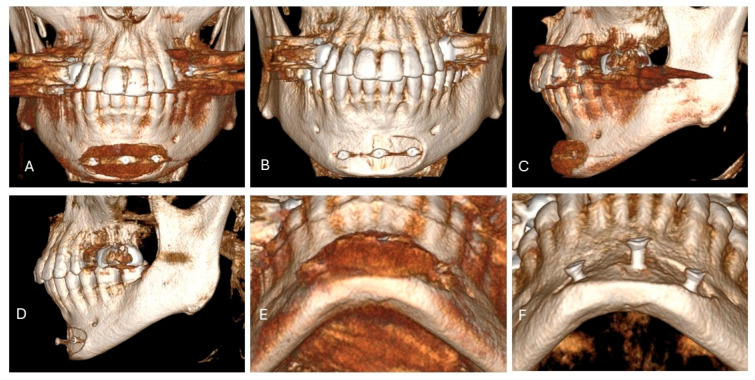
A 31-year-old Caucasian man presented for consultation because he was dissatisfied with a previously placed stock silicone chin implant stabilized with titanium screws (A–F). Although the patient had undergone comprehensive dental and orthodontic treatment, he had a “weak” mandible and jawline from untreated Class II malocclusion. CT showed that, despite bony fixation with titanium screws, the implant had caused mild bone resorption at the implant interface (B,D,E). The treatment plan called for removal of the silicone implant and screws, followed by immediate masculinizing genioplasty and placement of custom mandibular angle implants according to our protocol for lower-third masculinization.
